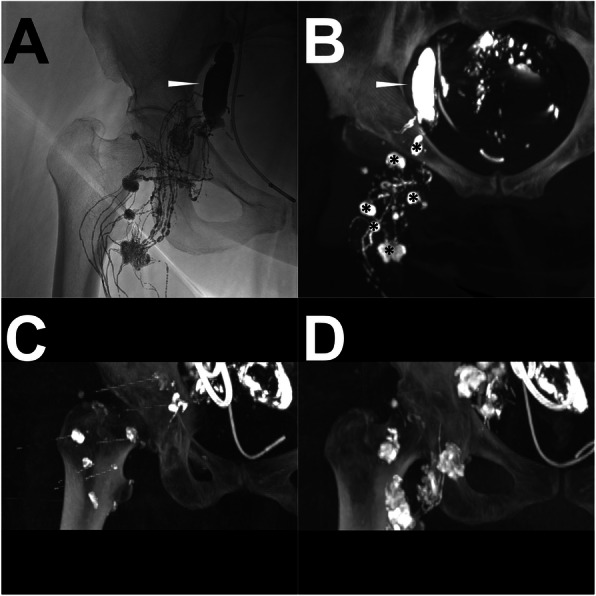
A patient underwent a TNE procedure after post-LAG CT. Note: The conventional iodized oil-based LAG shows the obvious extravasation (white arrowhead) from multiple afferent LVs and LNs in the right pelvis (a). One (1) hour later, the post-LAG CT (b) was sequentially performed which shows similar findings (white arrowhead) and identifies seven definite afferent LNs (*). The TNE was carried out with a CT-controlled percutaneous puncture of seven afferent LNs (c). After the embolization with the histoacryl/lipiodol mixture (1:1), the optimal distribution of the histoacryl/lipiodol mixture in afferent LNs and LVs is observed (d). Abbreviations: TNE – trans-afferent nodal embolization; post-LAG CT – post-lymphangiographic computed tomography; LAG – lymphangiography; MIP – maximum intensity projection; LVs – lymphatic vessels; LNs – lymph nodes
